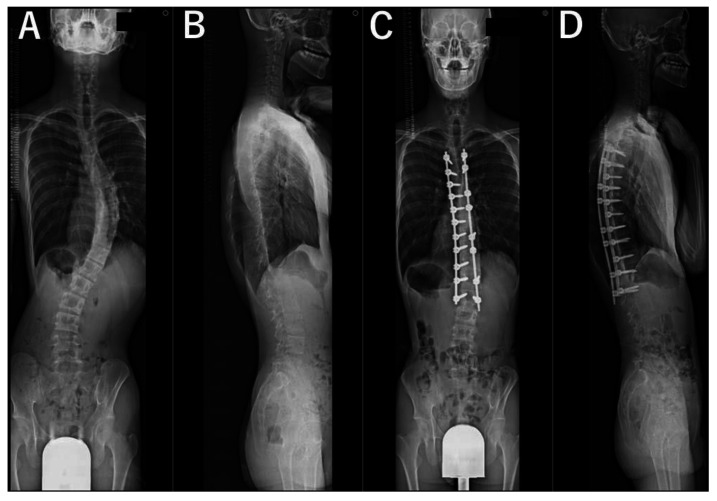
Pre- (A,B) and post- (C,D) operative radiographs of representative case with 16-year-old male patient. Cases of preoperative cervical kyphosis changed to postoperative cervical lordosis. Cervical lordosis (−5°, 18°), thoracic kyphosis (12°, 15°), lumbar lordosis (48°, 42°), T1 slope (11°, 22°), major thoracic curve (53°, 18°). (Preoperative, postoperative).
